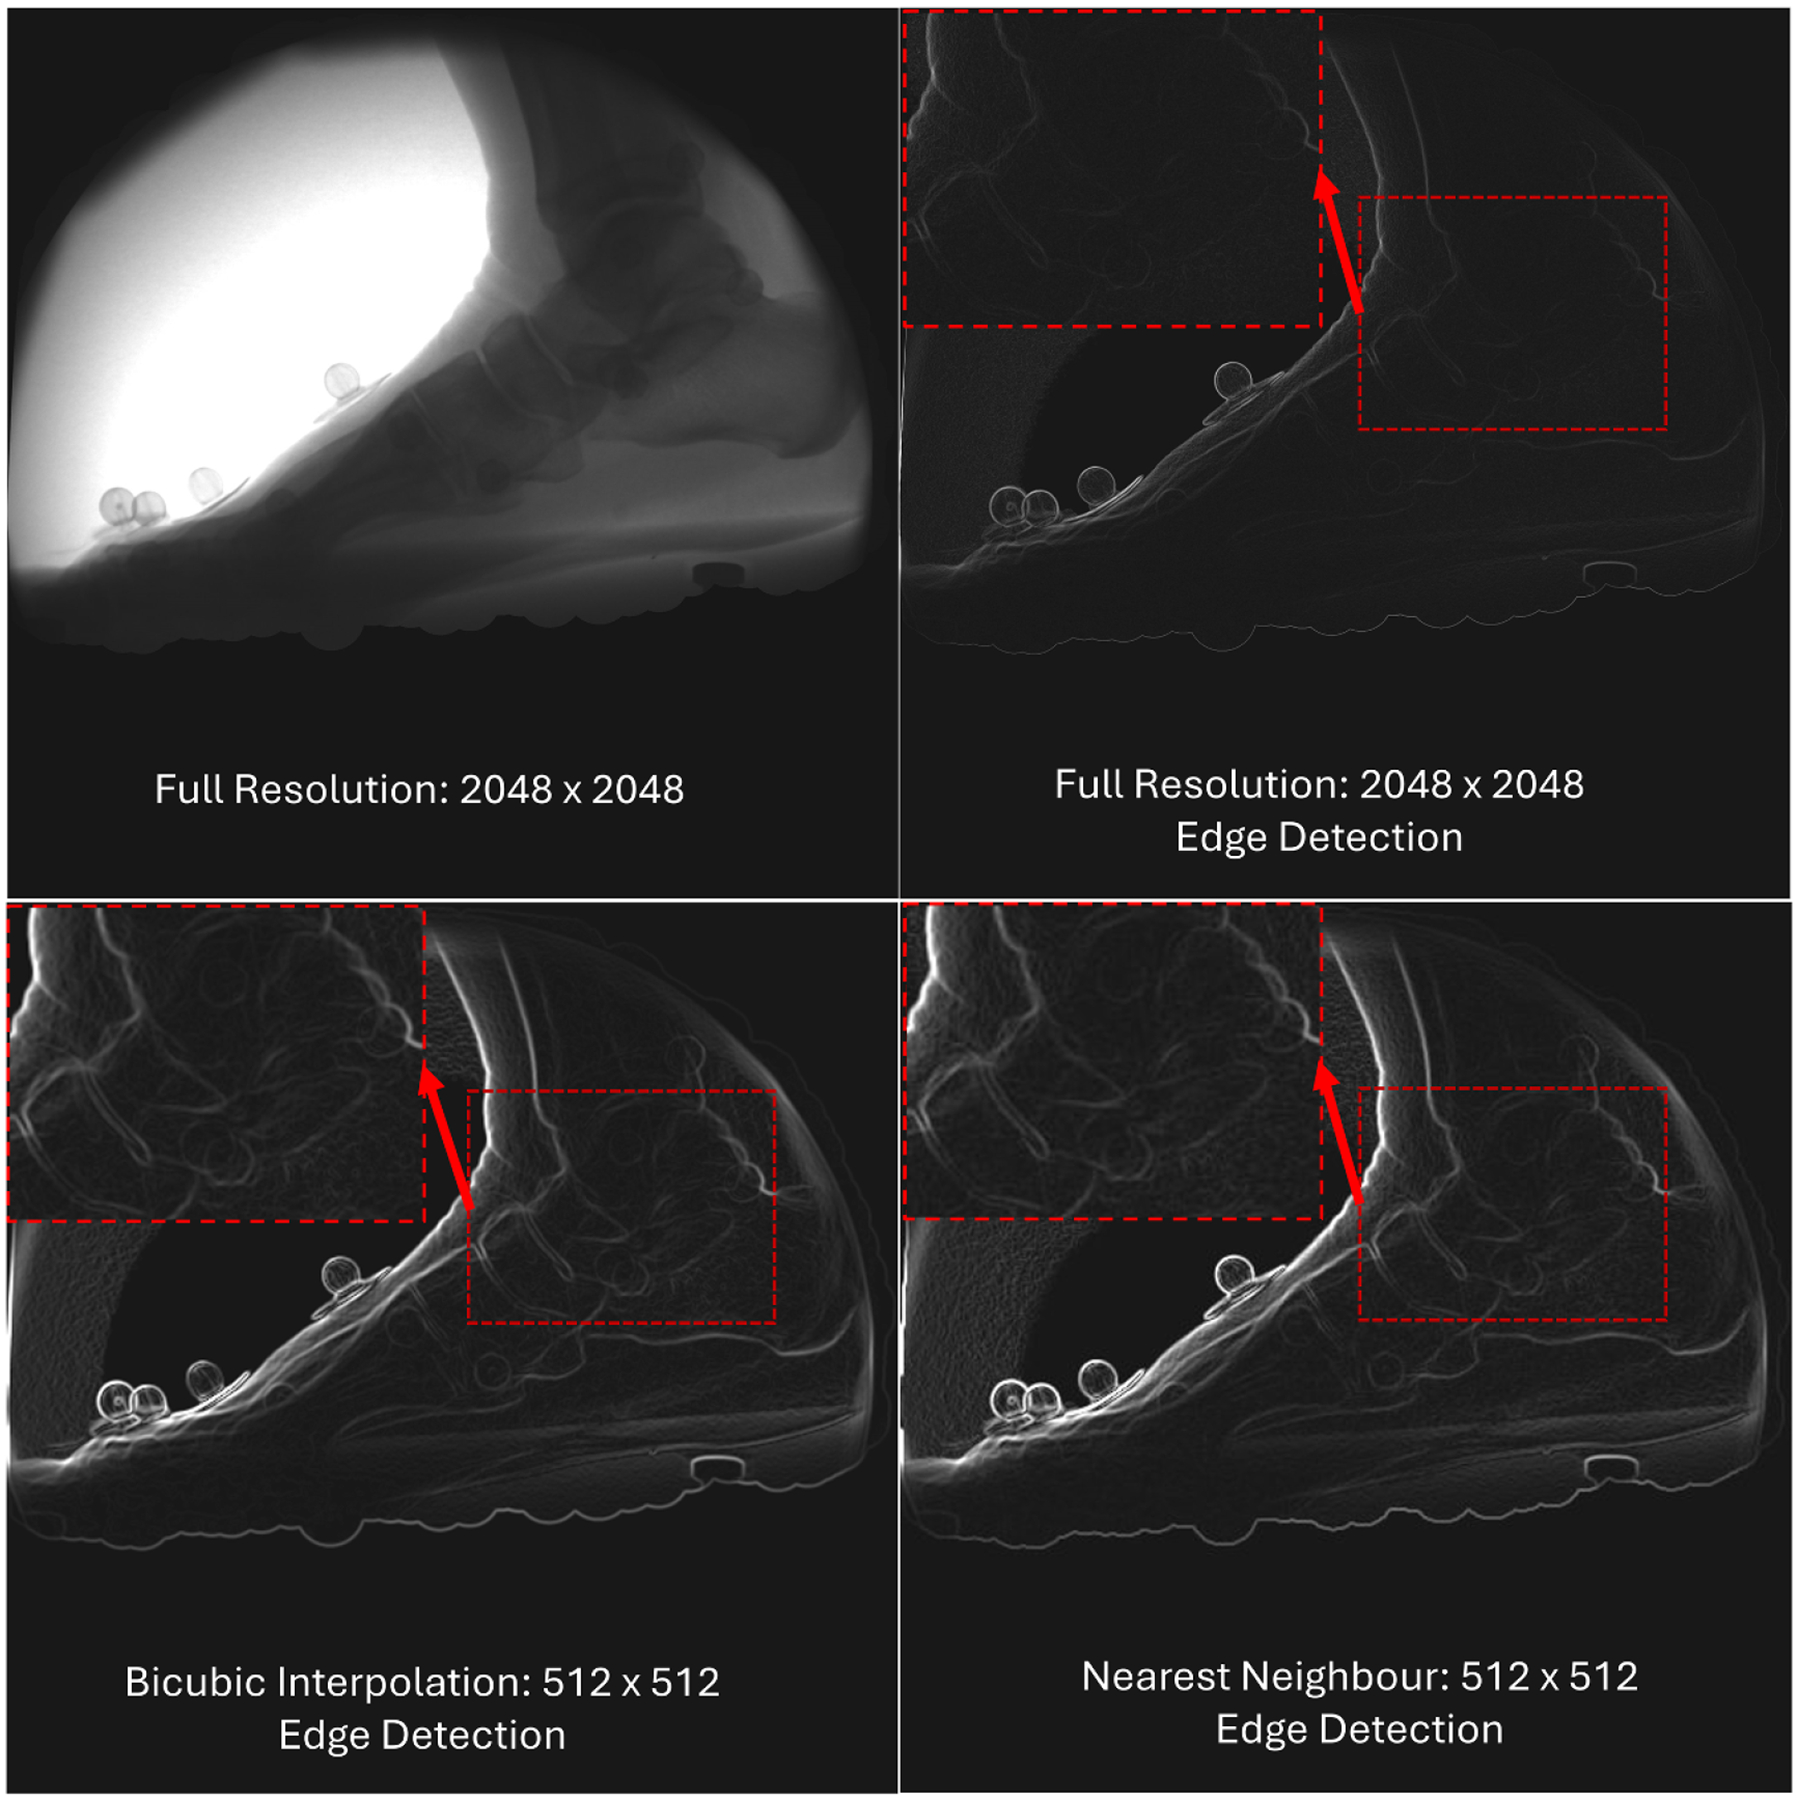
X-ray of foot during activity at different resolutions and edge detection applied. The bottom images show the downsampled images have stronger lines compared with the full resolution (top-right).
